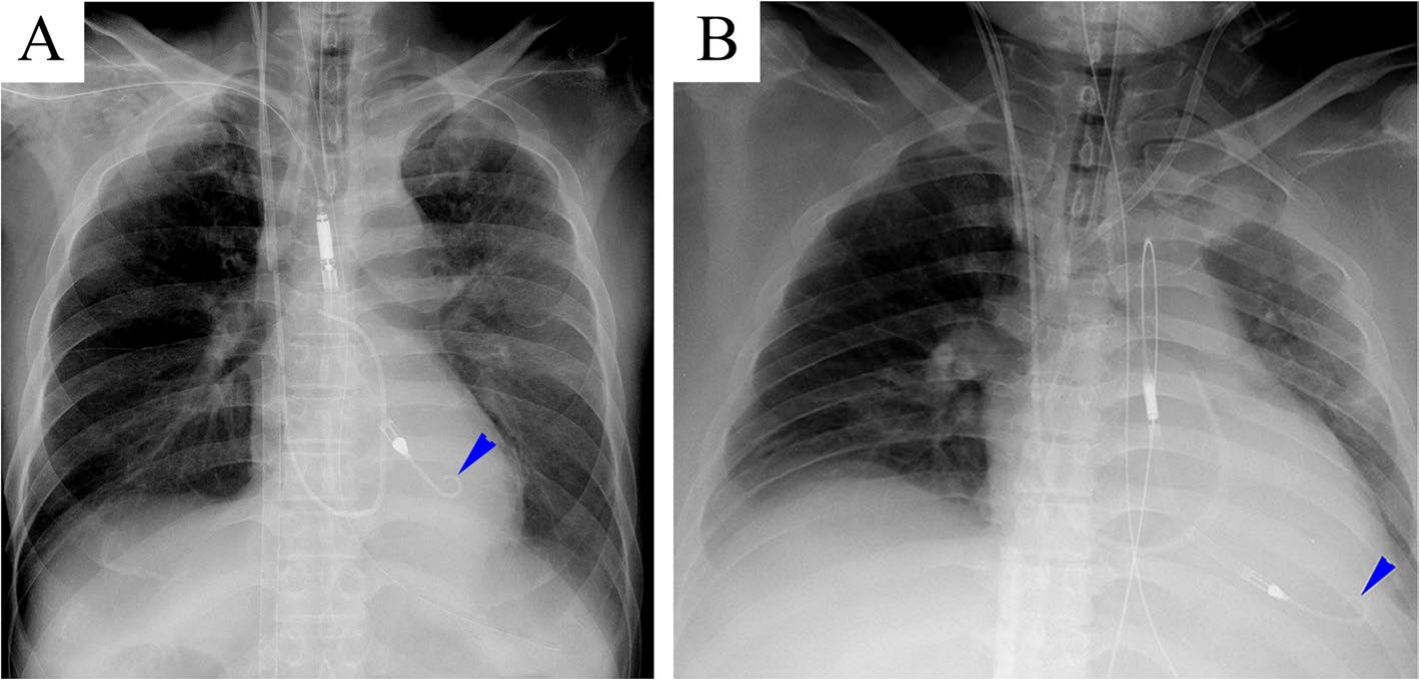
Supine chest x-rays in two cases. A A supine chest x-ray in a 67-year-old man who presented with fulminant myocarditis on the left side. The blue arrowhead indicates the tip of the Impella 5.0 indwelled via a vascular prosthesis surgically anastomosed to the right subclavian artery. B A supine chest x-ray in a 49-year-old man presented with fulminant myocarditis on the right side. The blue arrowhead indicates the tip of the Impella CP indwelled percutaneously via the left femoral artery
